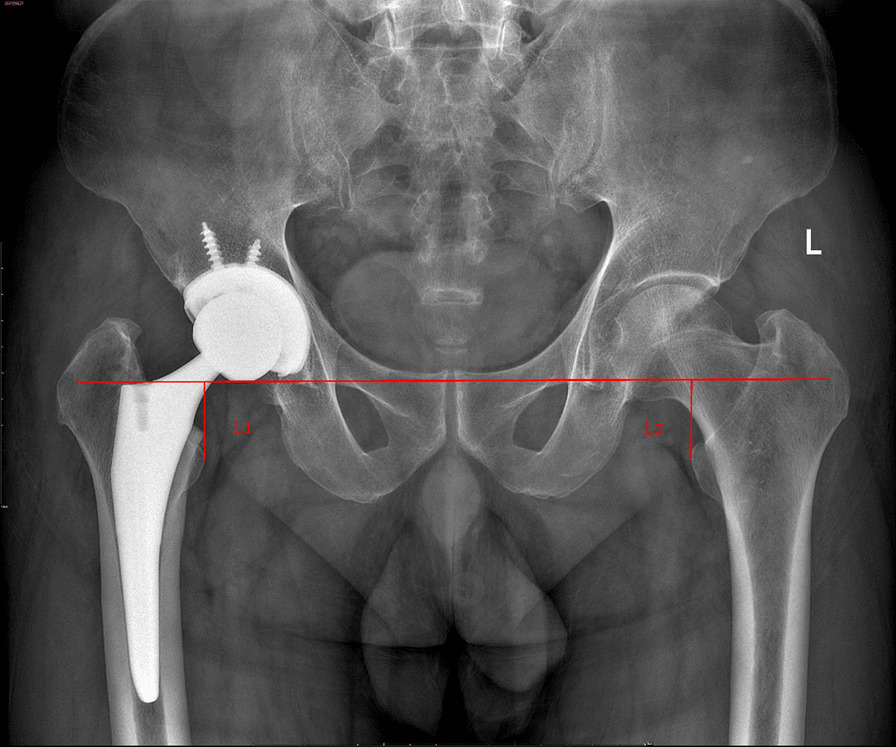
An AP radiograph shows the radiographic measurements of LLD in a male patient. The inter‐teardrop line was used as a pelvic reference. The two lines were drawn, each perpendicular to the teardrop line, starting from the most prominent portion of the lesser trochanter. LLD was defined as the difference in measurement between the operated and reference hip (L1–L2)
